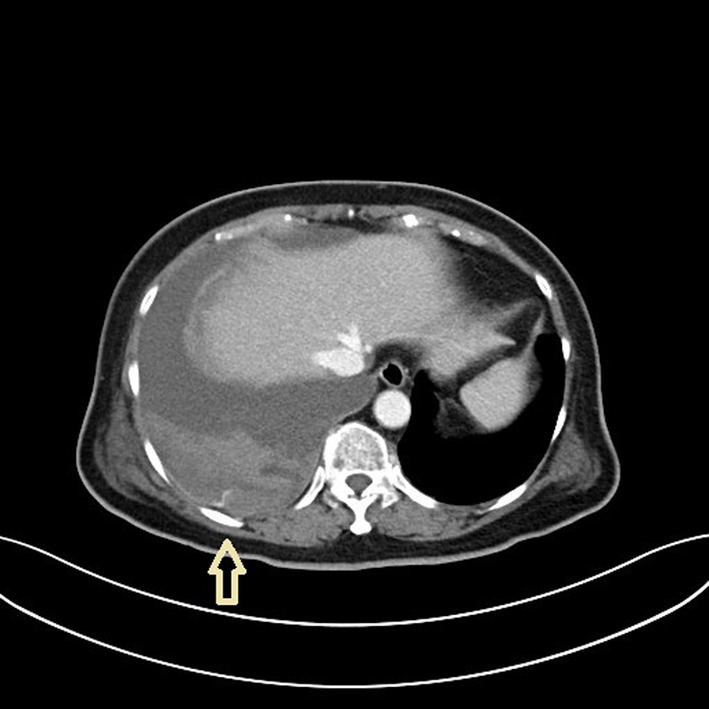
Computed tomography showed right‐sided pleural effusion and contrast medium extravasations from the right intercostal artery (arrow)
